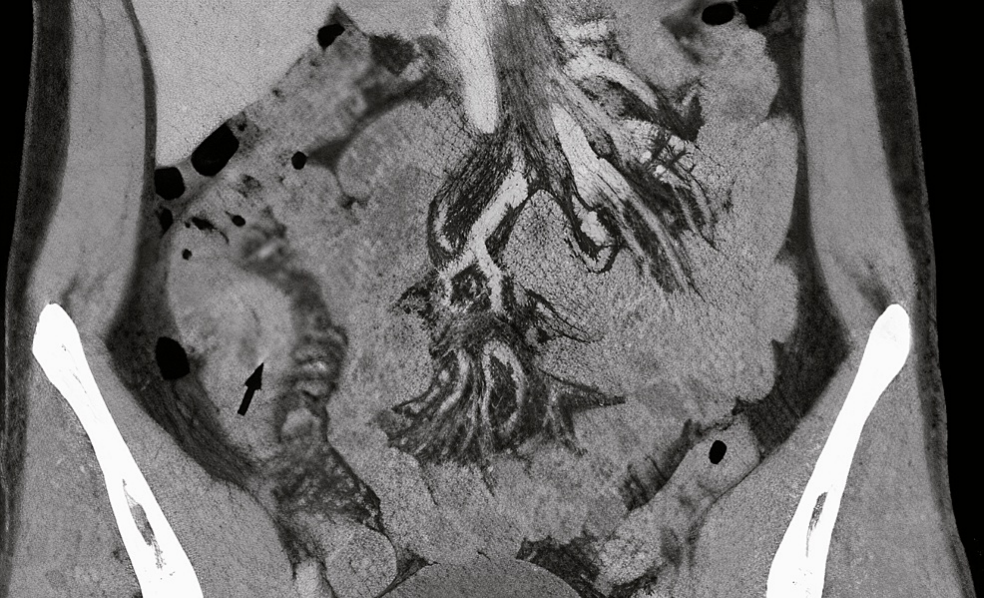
CT scan showing diffuse long segment mucosal thickening in the distal ileum, which extended over more than 12 cm. Arrow shows several enlarged lymph nodes on the right side of the bowel.
